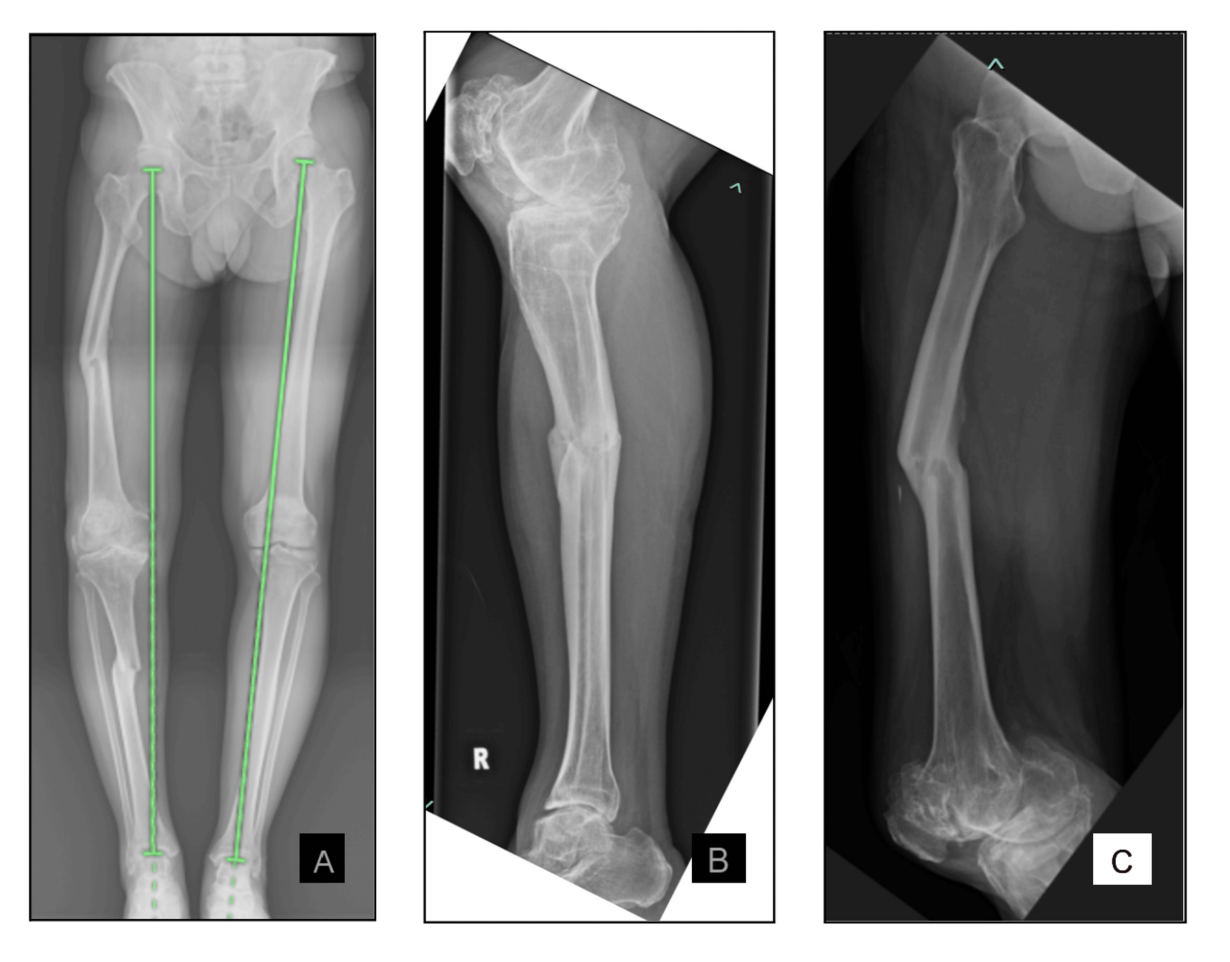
Preoperative radiographs. A) Bilateral lower limb scanogram of the anterior-posterior view, B) right tibia lateral view, and C) right femur lateral view Figure 1A shows the bilateral lower limb alignment during standing. This figure shows the coronal plane deformities of the femur and tibia shaft. The green lines indicate the mechanical axis of each lower limb. There is a deviation in the mechanical axis of the right lower limb. The right knee joint has severe osteoarthritic changes. Figure 1B is a lateral view of the right tibia showing the deformity of the tibia in the sagittal plane. Figure 1C shows the lateral view of the right femur showing the deformity of the femur in the sagittal plane. The lateral knee view of the right knee joint is also visualised with osteoarthritic changes.
